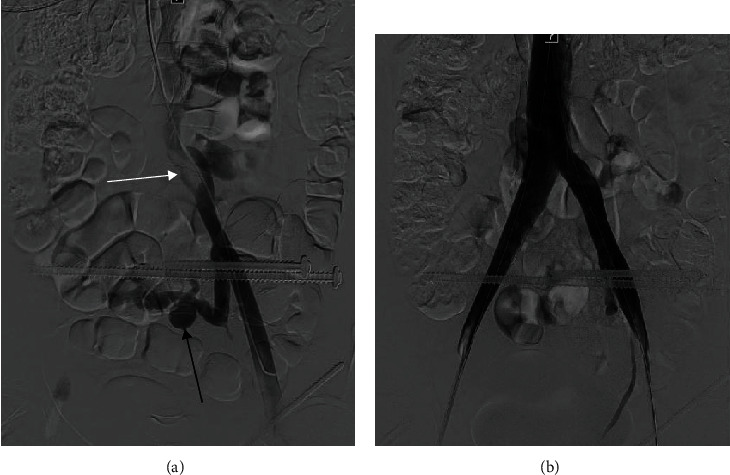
(a) Digital subtraction venography image demonstrating compression and narrowing of the left common iliac vein (white arrow) and a large venous pseudoaneurysm arising from pelvic varicosities (black arrow). These findings correspond with those seen on CT in Figure 1. (b) A digital subtraction venography image demonstrating the anatomic flow of contrast through the bilateral “kissing” iliac vein stents with resolution external compression of the left common iliac vein and associated narrowing. Also note that the pelvic varicosities and associated pseudoaneurysm are no longer visualized.
